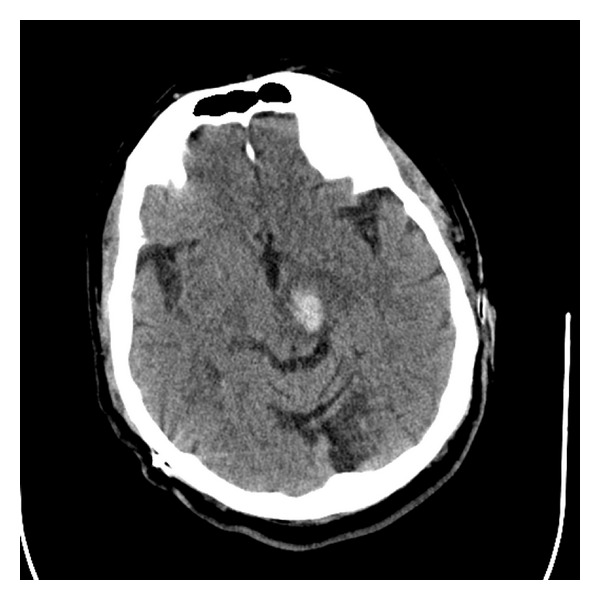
Repeat axial head CT without contrast enhancement done 24 hours after initial CT scan showing interval improvement of left cerebral peduncle hemorrhage.
